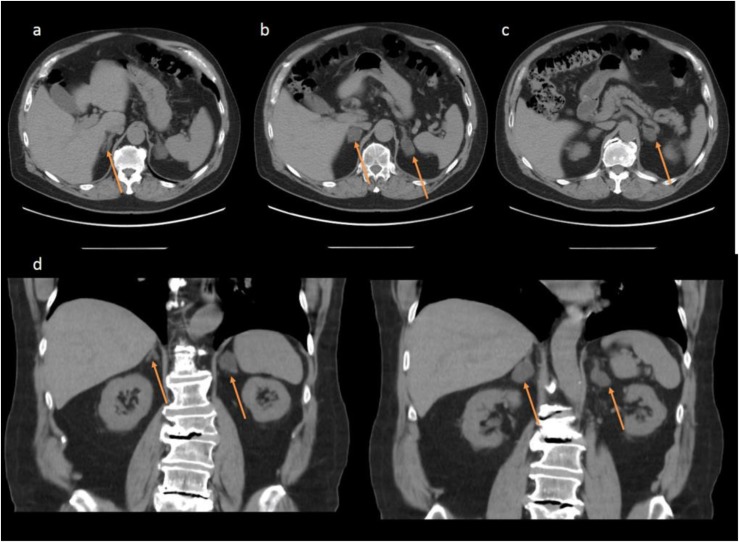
CT images from case 3 demonstrating bilateral adrenal nodules. (a–c) Multiple axial views of adrenal nodules, indicated by arrows. (d) Coronal images of bilateral adrenal nodules (arrows).
